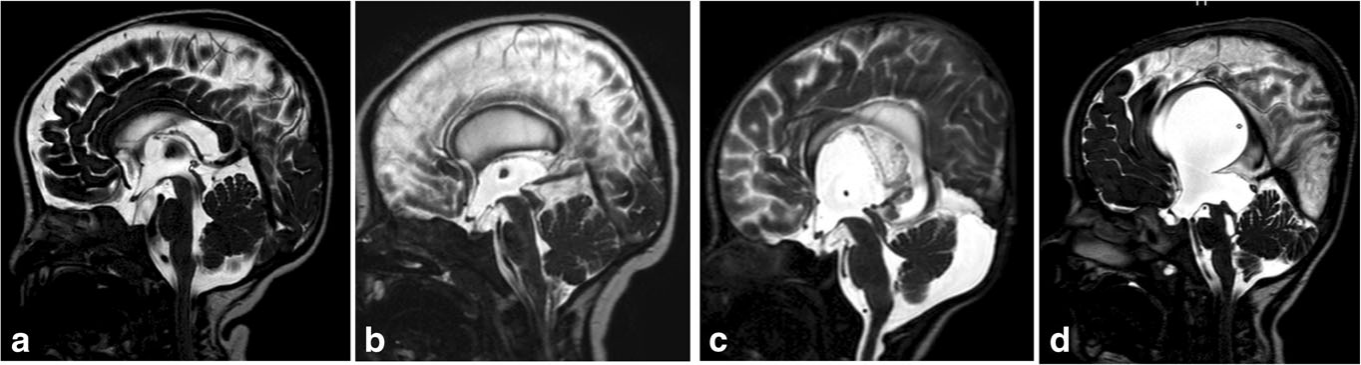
Midsagittal 3D-DRIVE showing the aqueduct of Sylvius and third ventricular anatomy in a normal infant (a) and patients with aqueduct stenosis (b–d). a A normal third ventricle of a 1-year-old girl. The lamina terminalis and tuber cinereum are concave centrally which may suggest that the mean pressure in the third ventricle may be lower than that in the cisterns. Note the signal void within the patent aqueduct of Sylvius. b MRI of a 3-month-old with mild hydrocephalus showing dilatation of the third ventricle recesses with bulge of the lamina terminalis secondary to multifocal aqueduct stenosis. c An 8-month-old girl with hydrocephalus secondary to congenital aqueduct stenosis (note the proximal funneling) showing more dilatation of the third ventricle recesses and more bulge of the lamina terminalis and third ventricle floor. d A 5-year-old boy with severe hydrocephalus with effacement of the chiasmatic and suprasellar recesses of the third ventricle. Note dilatation of the pineal recesses
